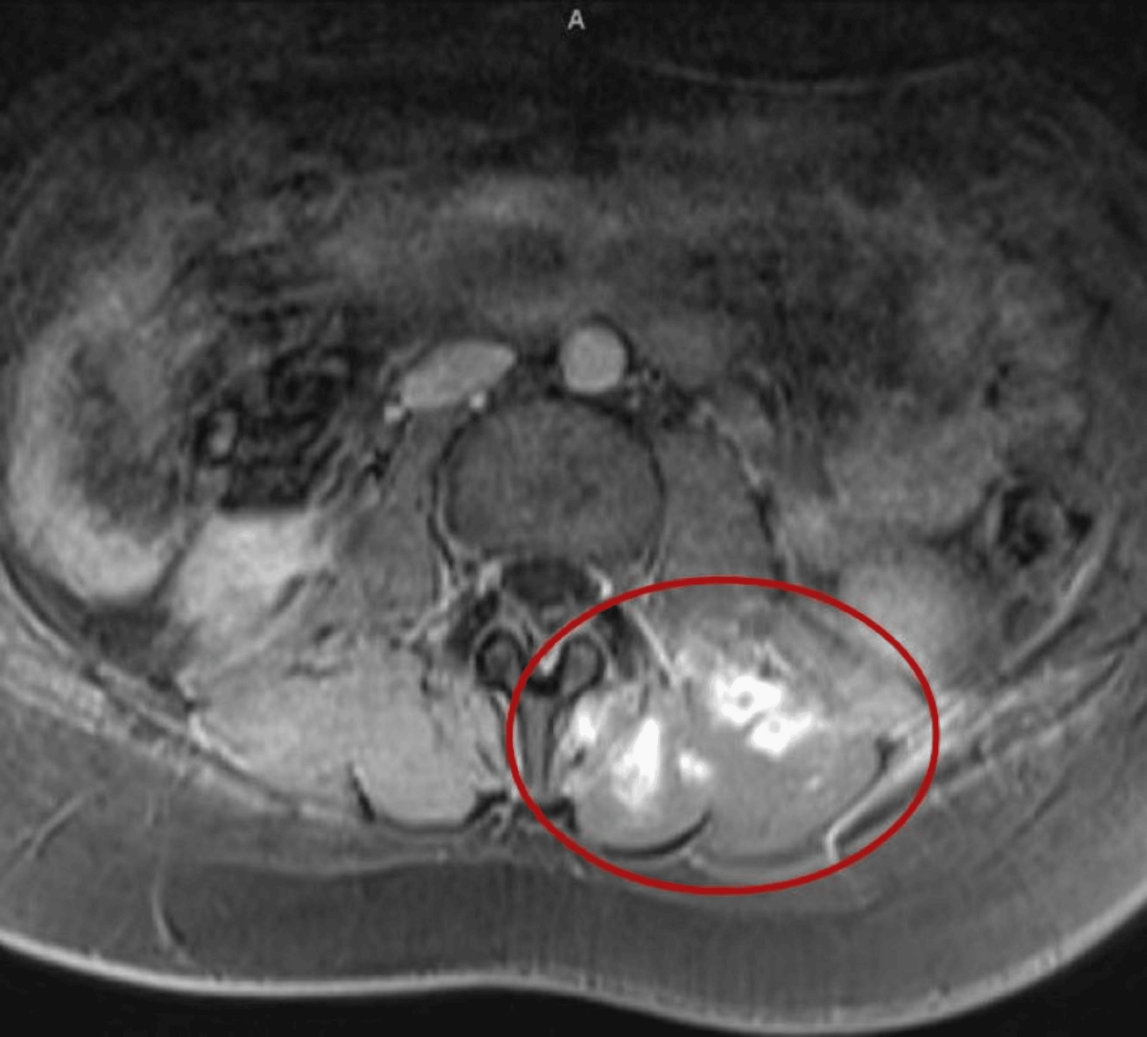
Axial T2-weighted MRI of the lumbar region showing multiple ring-enhancing nodular enhancing lesions consistent with pyomyositis. Axial T2-weighted MRI of the lumbar spine and posterior abdominal region demonstrates multiple ring-enhancing nodular lesions (highlighted within the red circle) involving the left multifidus, erector spinae, and quadratus lumborum muscles. These findings are consistent with focal areas of inflammation, edema, and soft tissue involvement, which are representative of pyomyositis. MRI: magnetic resonance imaging.
